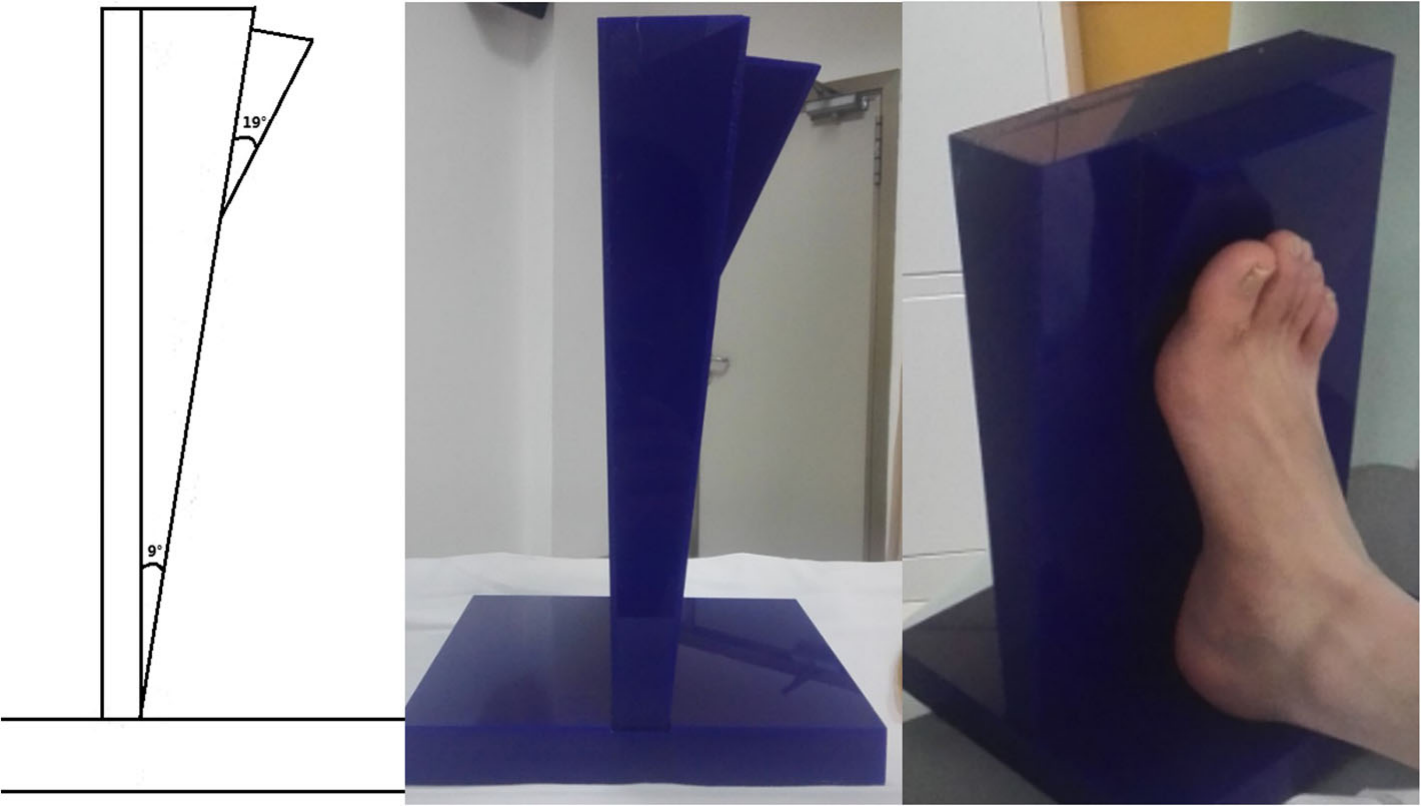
Customized foot plate used to maintain the joints in position during CT scans. As shown in the figure, the dorsiflexion of its ankle and MTP joint was controlled at 9 degrees and 19 degrees respectively
